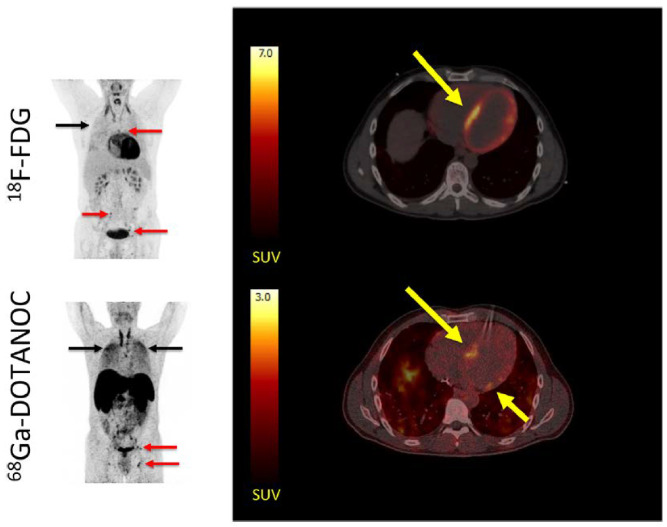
MIPs (left) showing multiple ^18^F-FDG and ^68^Ga-DOTANOC avid lymph nodes (red arrows) and lung tissue (black arrows) in a patient with both cardiac and systemic sarcoidosis. Transaxial slices of the heart (right) showed a focal on diffuse pattern of ^18^F-FDG uptake (top right), which was rated as inconclusive by all reviewers, compared to all reviewers rating the ^68^Ga-DOTANOC uptake (bottom right) as pathologic in the septum. Reprinted from ref. [65].
